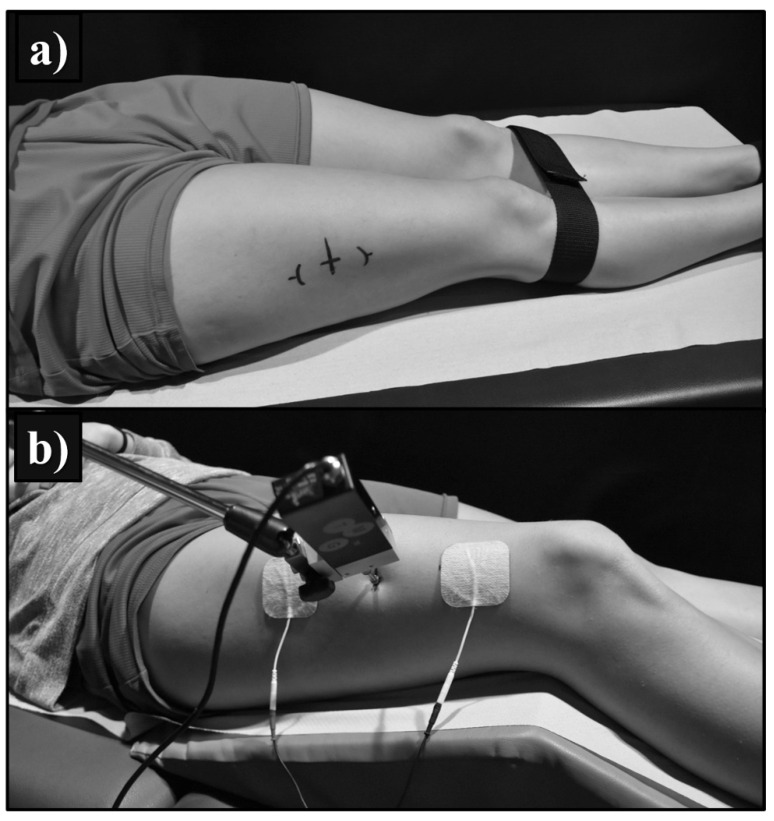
Position of participants during assessments. (a) Measurement position of ultrasound and MyotonPRO exams of Vastus Lateralis. (b) Measurement position of tensiomyography exam of Vastus Lateralis.
